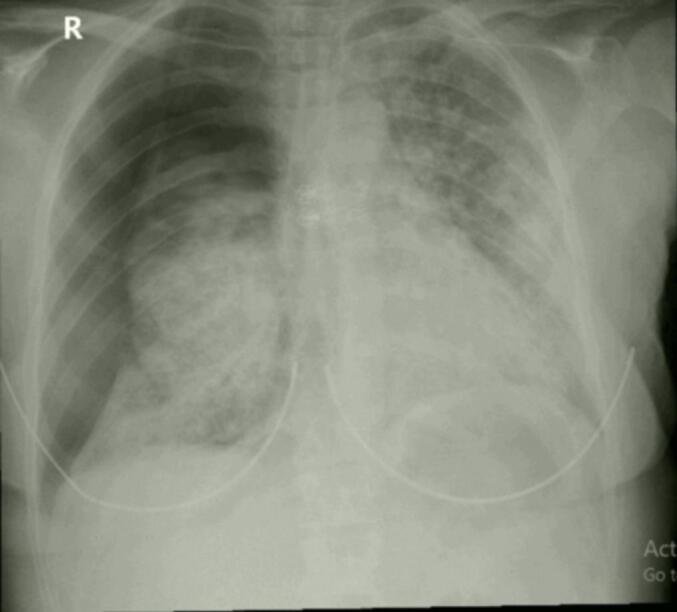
chest X-ray (CXR) showing a right pneumothorax, prominent diffuse bilateral interstitial alveolar opacities.
